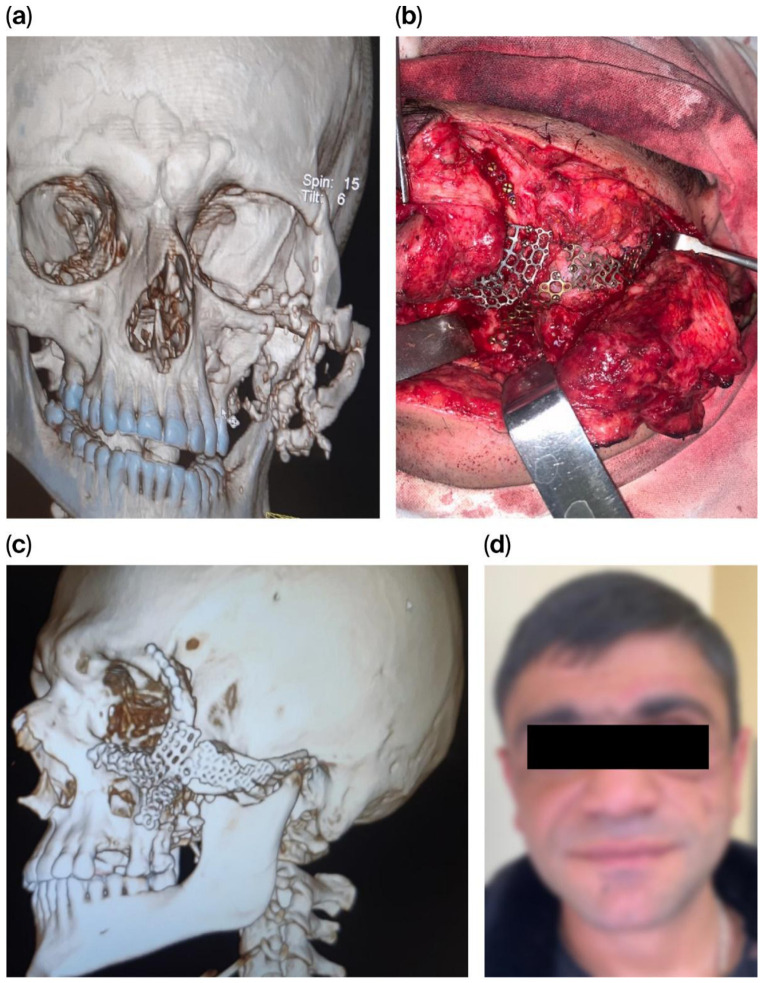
Drone-blast midfacial injury: (a) 3D CT scan demonstrates a left-site severely comminuted midface fracture; (b) early definitive reconstruction osteosynthesis using titanium mesh and plates; (c) postoperative 3D CT scan; (d) long-term (5-year follow-up) postoperative result demonstrating soft tissue thinning over titanium mesh and plates in the left zygomatic region.
